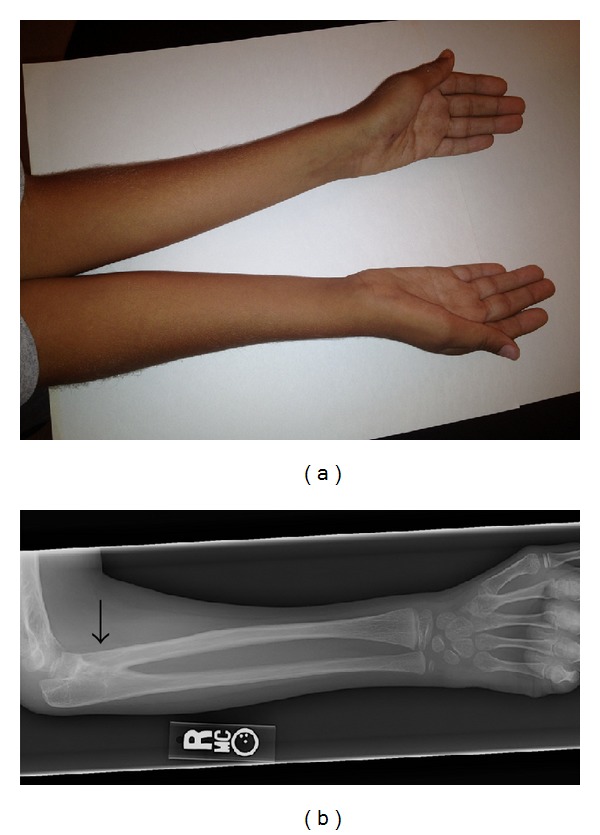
Photograph showing the patient's forearm with limitation of rotation (a). There is also hypoplasia of the thenar and hypothenar muscular prominences of his hands bilaterally. Radiograph of the right forearm (b). The arrow indicates the site of bony synostosis.
